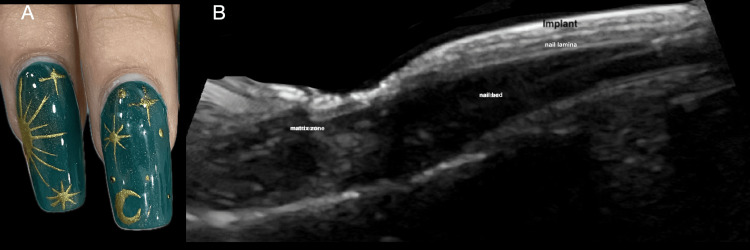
Ultrasound appearance of acrylic nails. (A) Clinical image of a patient with a manicure featuring acrylic nails. (B) Longitudinal high-resolution ultrasound image showing the acrylic nail implant as a moderately hyperechoic structure relative to the native nail lamina, located immediately above it.
